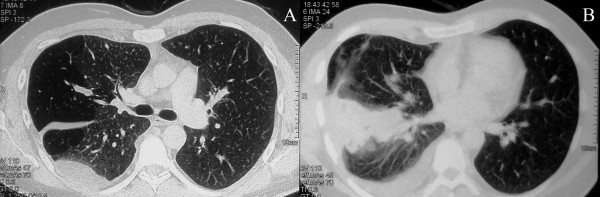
Chest computed tomography scan. (A) Areas of air-trapping with hyperlucency mainly in the right upper lobe (compatible with Swyer-James-MacLeod Syndrome). (B) A mass with pleural contact and a small pleural effusion in the right lung (semi-invasive aspergillosis).
